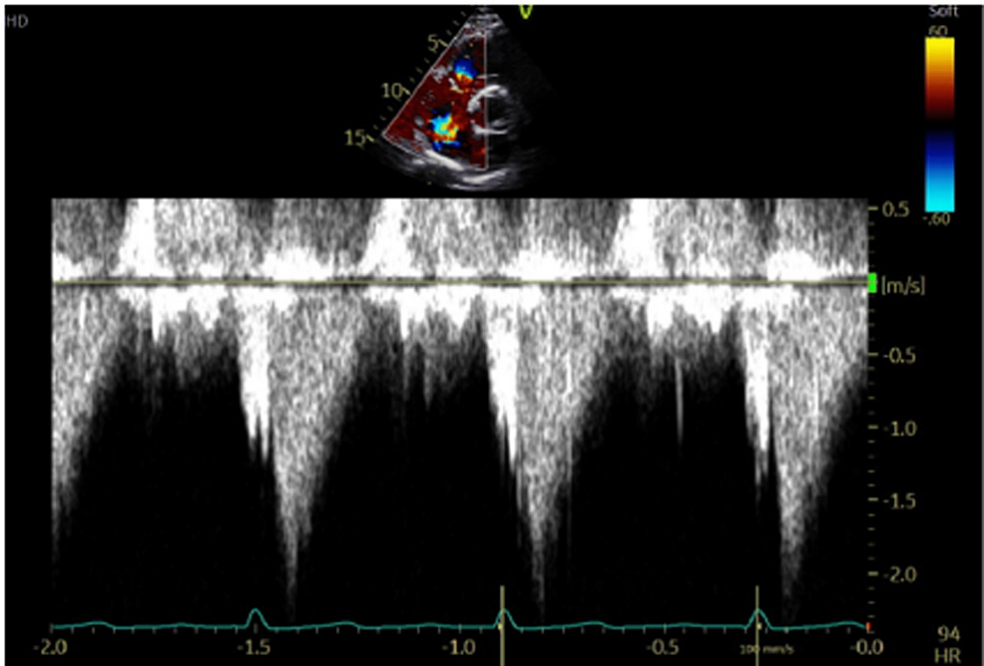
Continuous wave Doppler demonstrating a dense, triangular jet
